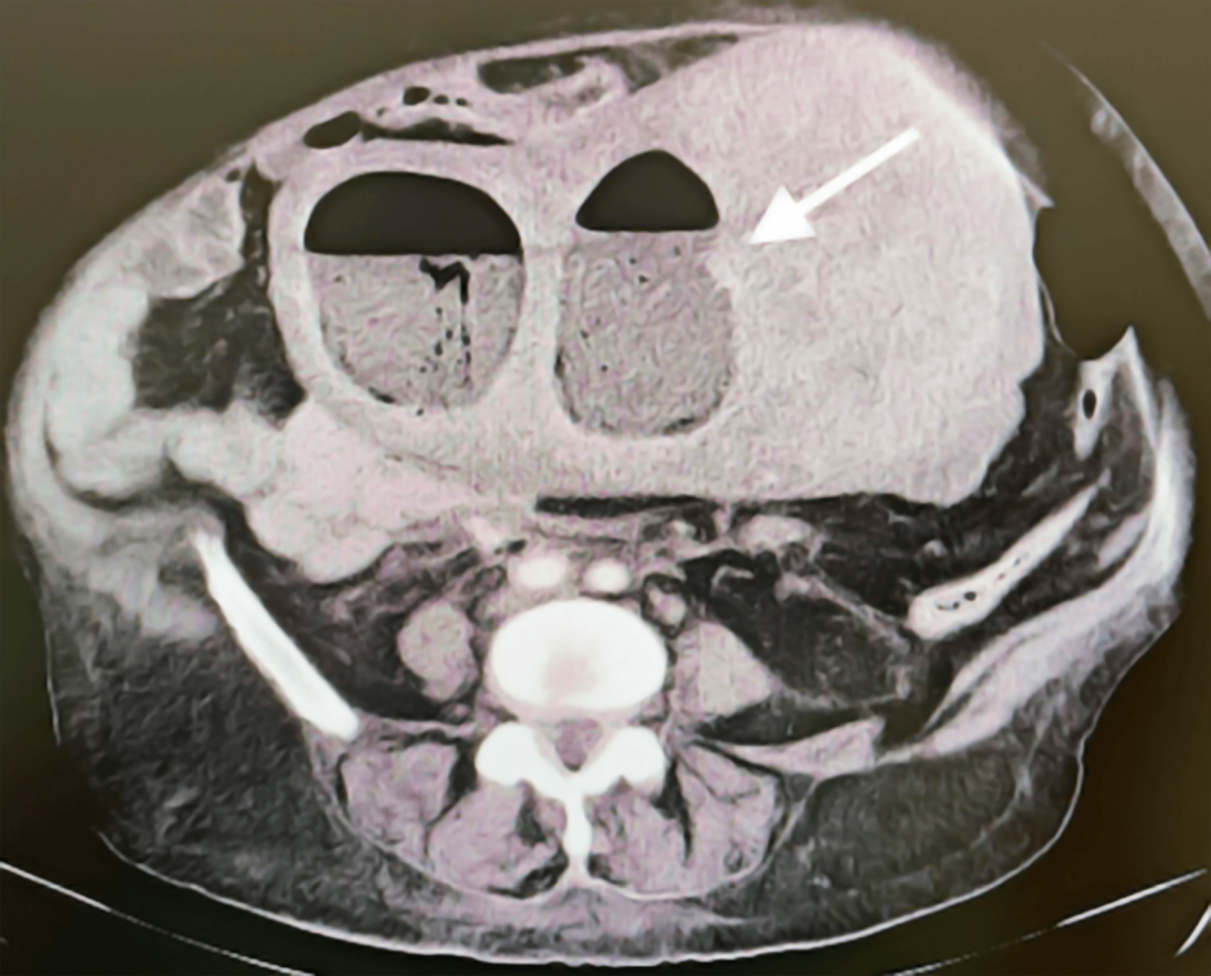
Abdomino-pelvic CT showing large intrauterine collection with air-fluid level and substantial solid-appearing nodular formation, consistent with a large hematopyometra.
